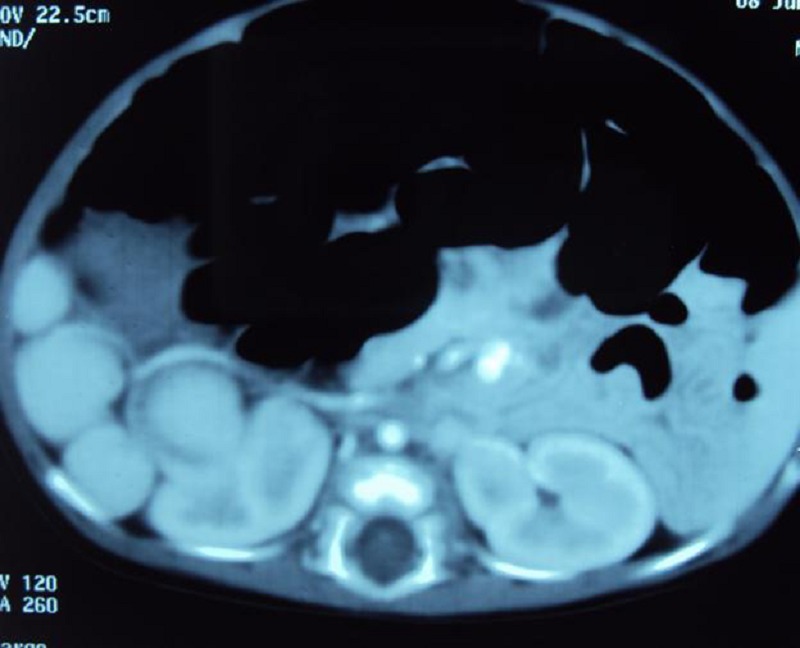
Figure 1: CT scan showing multiple splenicules.
